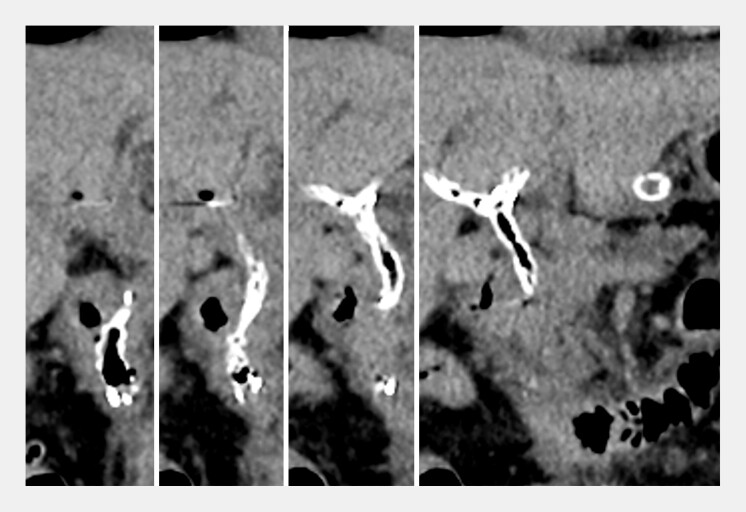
Computed tomography showing three SEMSs used in antegrade and bridging stenting, along with hepaticogastrostomy. SEMSs, self-expandable metal stents.
